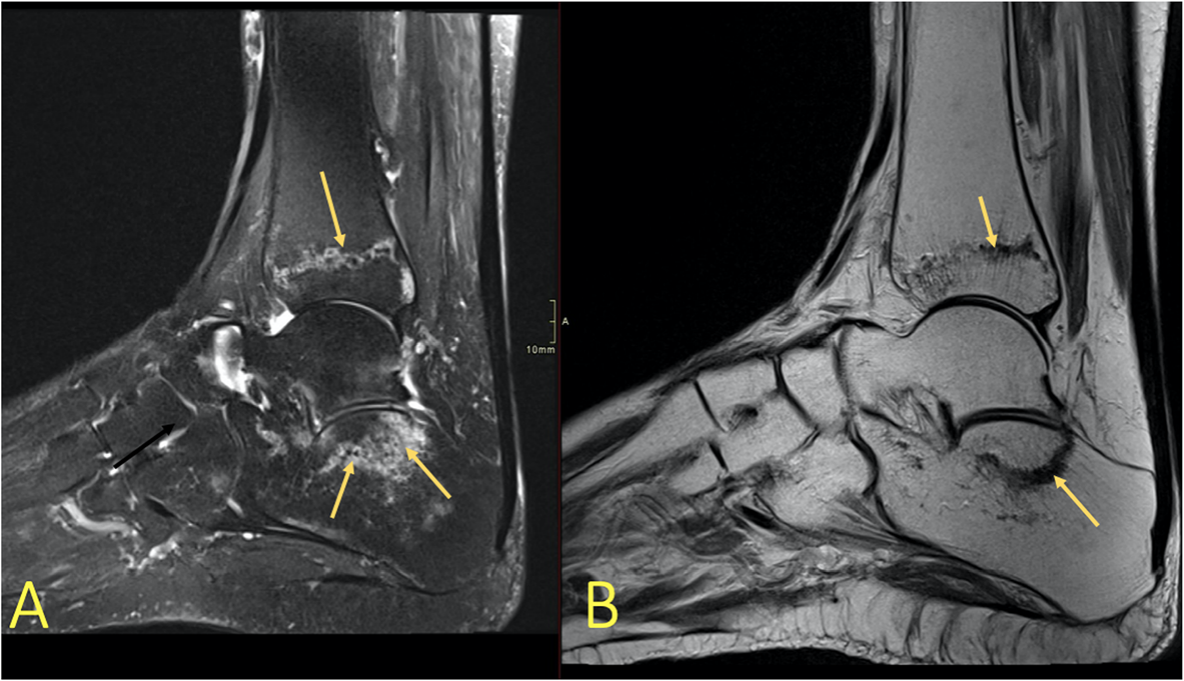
A 57-year-old female with hindfoot pain for 4 weeks and a suspected osteochondral lesion and osteoarthritis. MRI showed stress fractures of the tibia and calcaneus (arrows)
